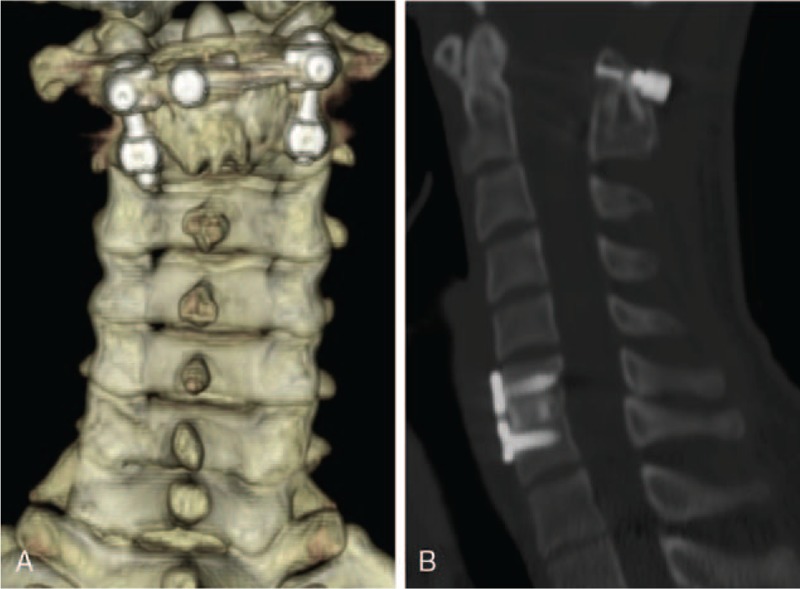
One-year postoperative computed tomography (CT) scans show solid union of C1–2 and C6–7.
